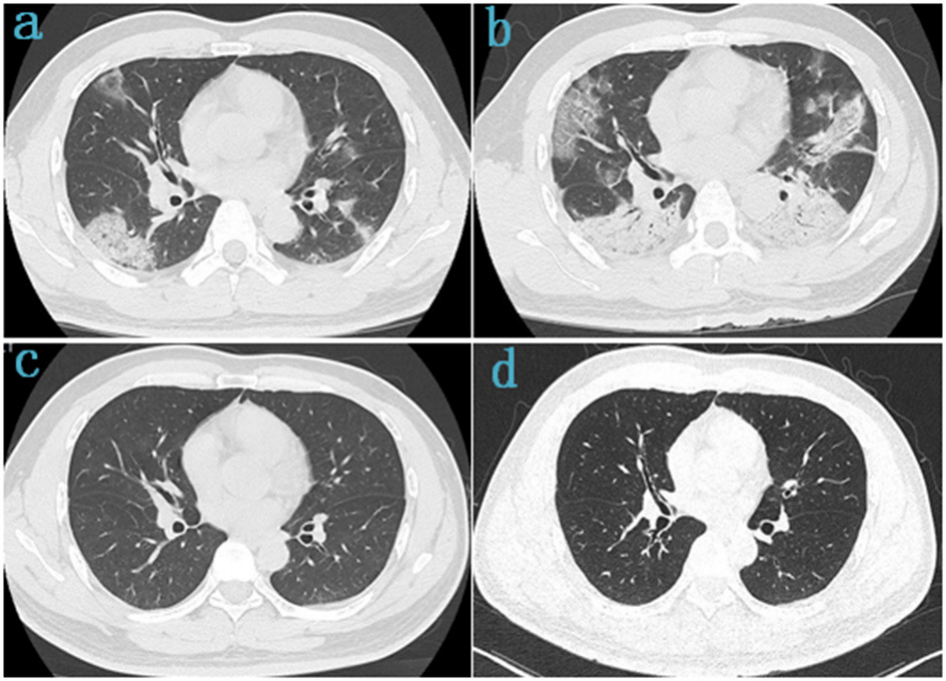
Chest CT of a 42-year-old male survivor of COVID-19 Delta variant. (a) On admission, baseline scan shows multiple bilateral ground-glass opacity with predominantly linear pattern and peripheral distribution, with air-bronchogram and tubular size increase of vessels in some lesions. (b) 4 days after admission, the lesions were significantly larger and more extensive than before, chest CT scans were subpleural ground-glass opacity that grew larger with crazy-paving pattern and consolidation. (c) Before discharge, the lesions in both lungs were basically absorbed. (d) At follow-up, the chest CT was basically normal, with a few fibrotic lesions were seen in the left lung.
